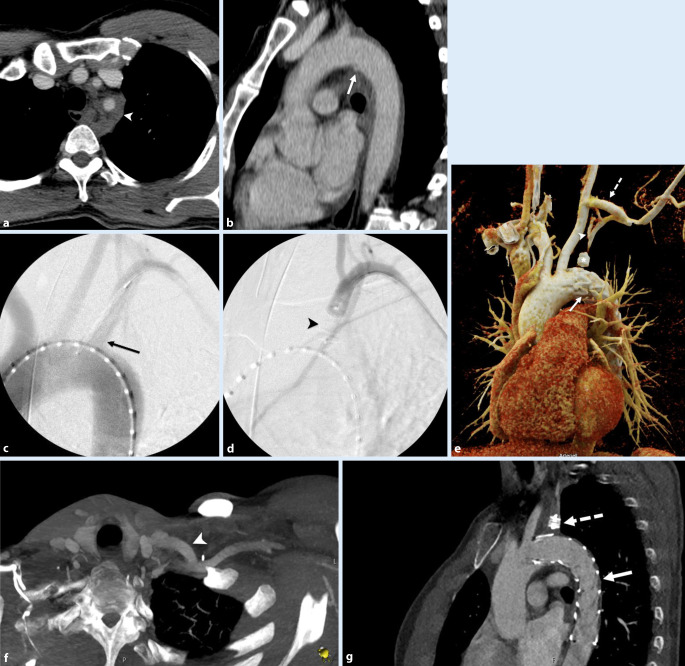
Auch andere Thoraxverletzungen können einen indirekten Hinweis auf eine TAV darstellen [31]. Abgesehen davon bietet bereits die native CT die Möglichkeit, ein akutes Wandhämatom von artheriosklerotisch-thrombotischen Wandauflagerungen zu unterscheiden [17]. Um diesen Effekt gut ausnutzen zu können, darf der Hämatokritwert des Patienten nicht zu niedrig sein (*Cave*: verdünntes Blut), und ein erfahrener Radiologe kennt auch weitere Unterscheidungskriterien auf Basis der KM-gestützten angiographischen Bildserie.
